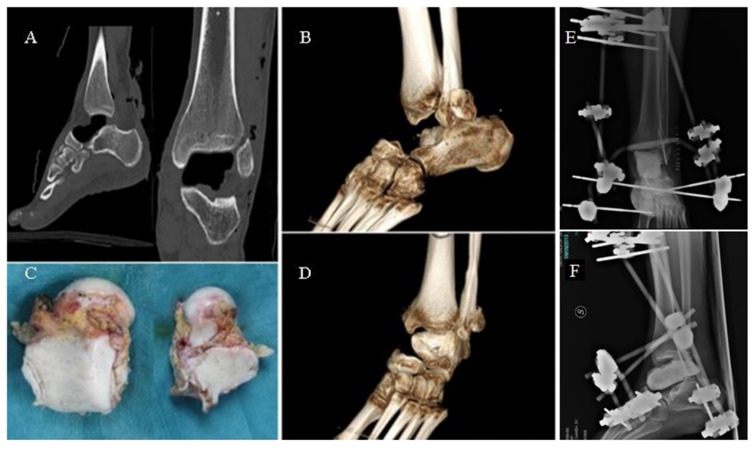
CT images (A) and volume rendering (B,D) showing the gap left by the talus (C). External fixation of the ankle joint (E,F).
